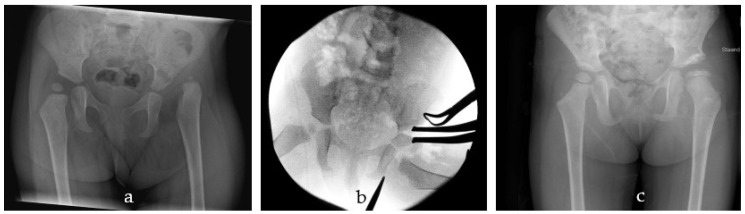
Radiographs of a Salter pelvic osteotomy. (a) Preoperative radiograph showing a late diagnosis of a dislocated hip in a girl aged 1 year and 9 months. (b) Intra-operative radiograph of pelvic osteotomy and open reduction at age 2 years. (c) Postoperative results after 2 years, showing postoperative avascular necrosis of the femoral head.
